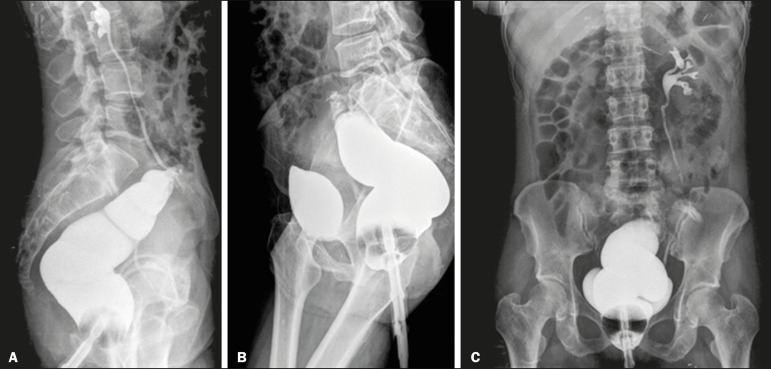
X-rays obtained after barium enema. A: Lateral view showing initial opacification of the rectum, remaining distal colon, ureter, and left renal collecting system. B: Lateral view showing late opacification of the bladder. C: Posteroanterior view showing late opacification of the bladder.
